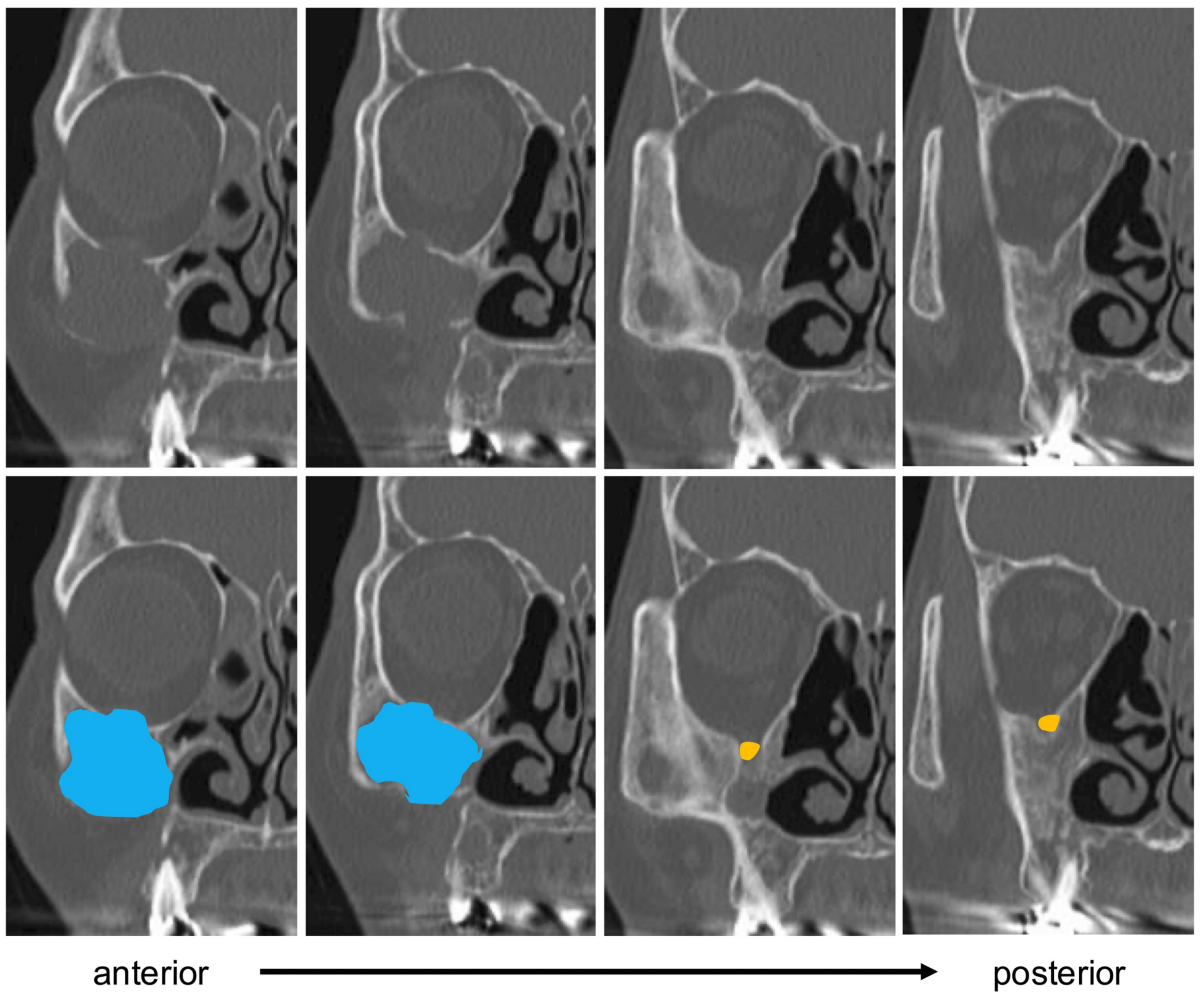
Preoperative computed tomography (CT) images The right side is anterior, and the left side is posterior as one moves toward the left. The upper panel shows the plain CT image, while the lower panel highlights the cyst in blue and the nerve in orange. It was not possible to identify the exact location of the infraorbital nerve.
